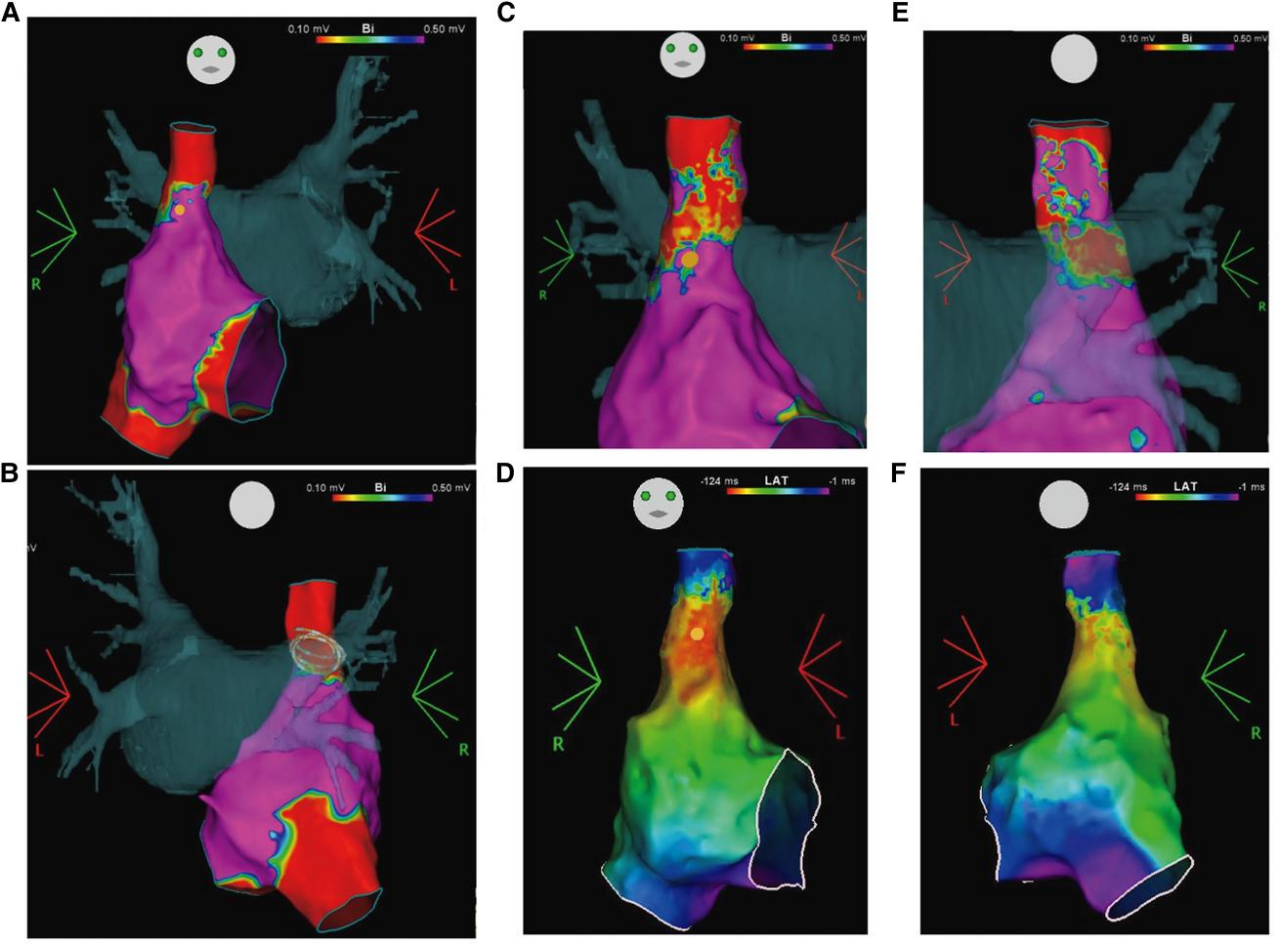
(*A* and *B*) Post-pulmonary vein isolation right atrial voltage map merged with left atrial computed tomography image. A low-voltage area was identified 5.8 mm from the superior border of the sinus node (yellow tag). Rings indicate pulsed field ablation application sites in the right superior pulmonary vein. (*C* and *D*) Right atrial voltage maps after pulmonary vein isolation, merged with left atrial computed tomography image, demonstrating dormant conduction. (*E* and *F*) Right atrial activation maps after pulmonary vein isolation, merged with left atrial computed tomography image, also demonstrating dormant conduction. The shortest distance between the sinus node (yellow tag) and the injured superior vena cava was 4.1 mm.
